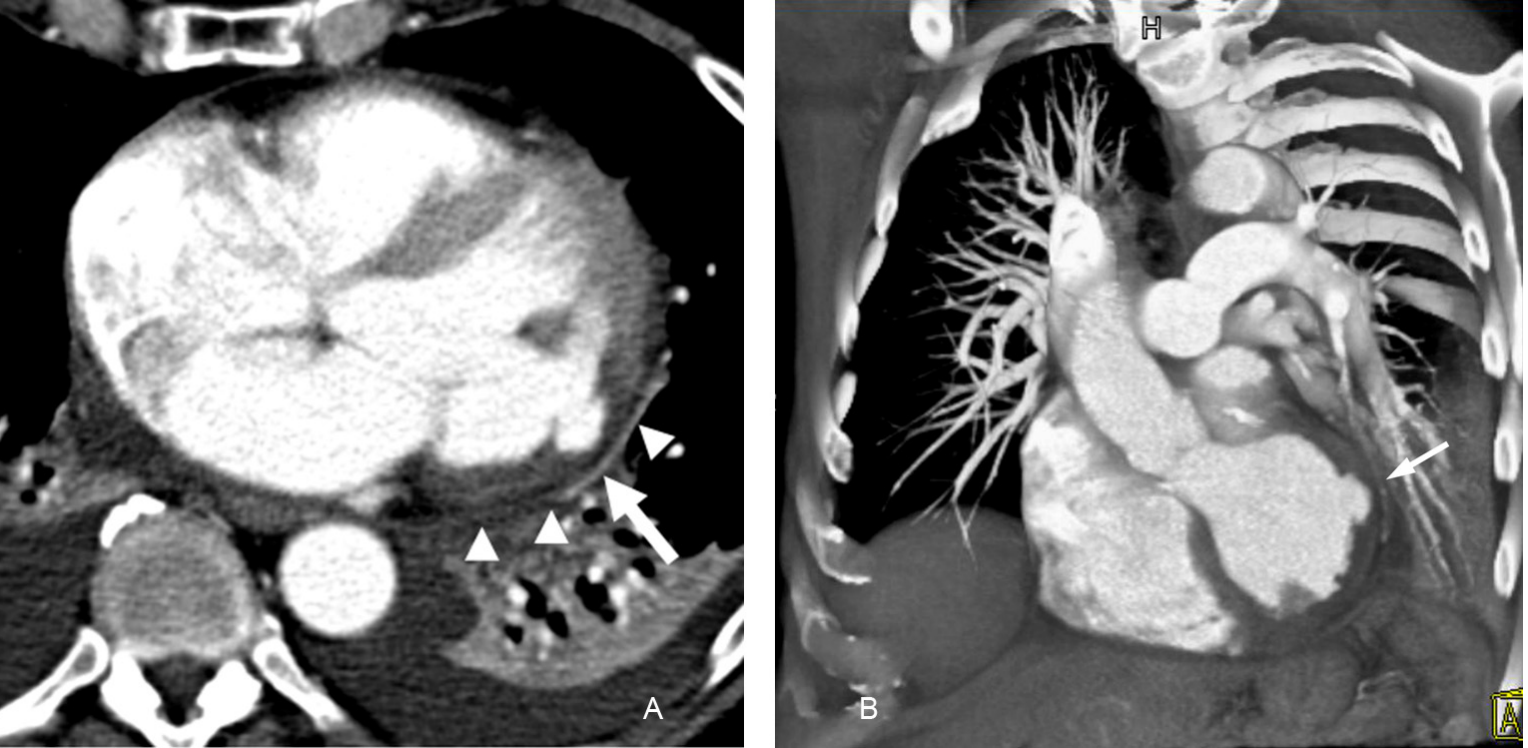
Axial contrast enhanced CT image of the chest (a) shows an area of decreased perfusion in the lateral wall of the left ventricle (arrowheads) with a 1 × 1.6 cm blister-like pouch (arrow). A volume rendered 3D MDCT image (b) of the left ventricle shows an area of localized contrast out-pouching with a narrow neck in the lateral left ventricular wall (arrow).
